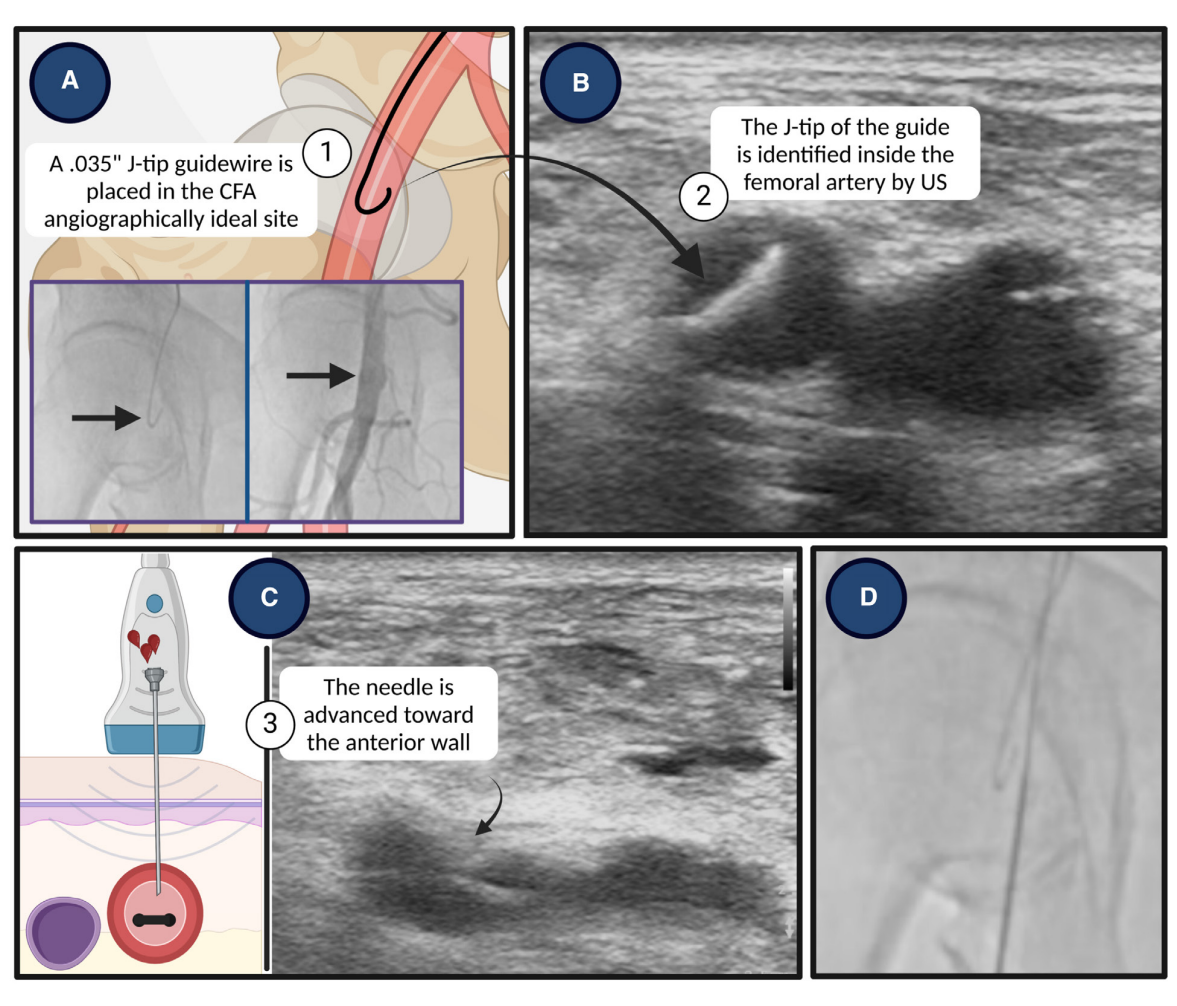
The angio-guided-ultrasound (AGU) guidance. CFA indicates common femoral artery; and US, ultrasound. Created with BioRender.com.
